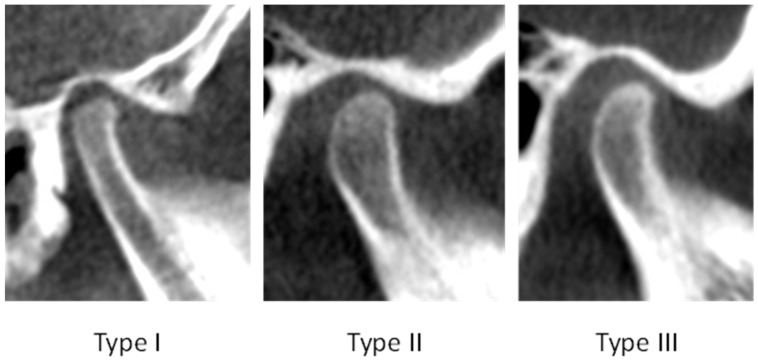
CBCT scans showing Type I, Type II and Type III cortication of the mandibular condyle.
